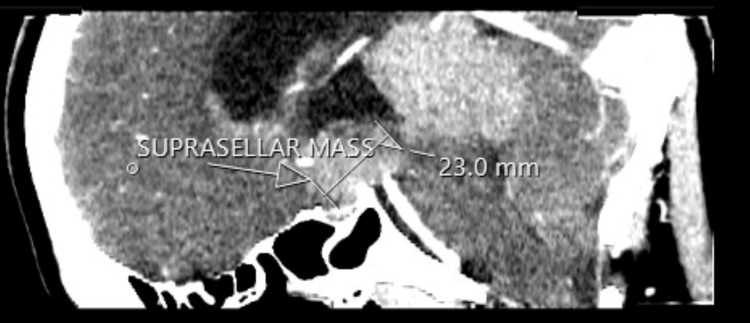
CT of the pituitary with contrast. White arrow: mass in the suprasellar region.
